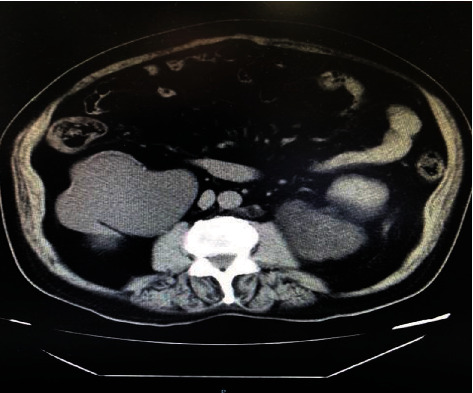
CT of November 2019 showing the important growth of the mass located between the left kidney and the psoas muscle.
